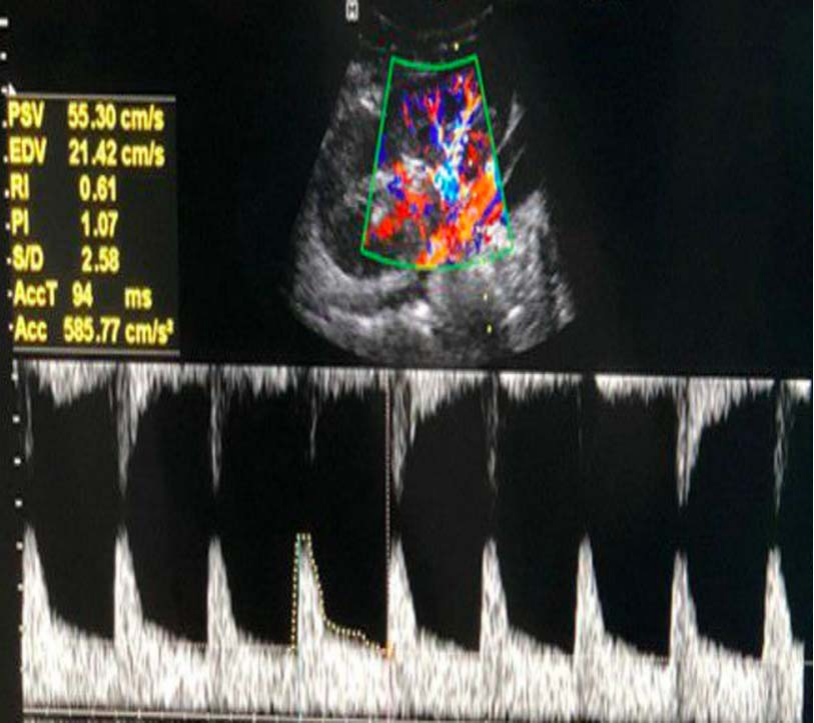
Spectral Doppler study showing the measurement of Peak systolic velocity (PSV) and Resistance index (RI).
